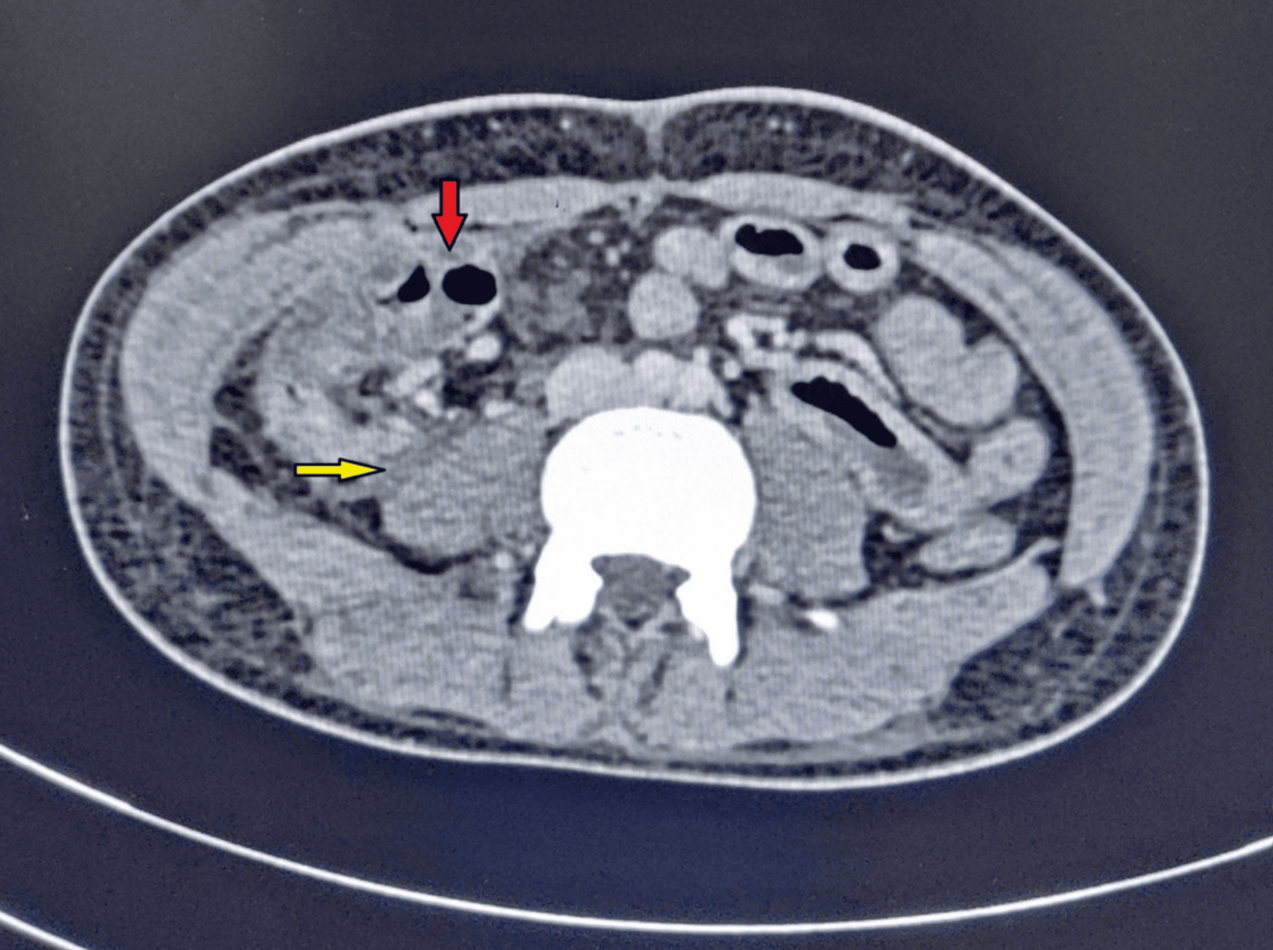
CECT of the whole abdomen showing anastomotic leak (yellow arrow) after ileo-ileal anastomosis (red arrow) CECT: contrast-enhanced computed tomography
